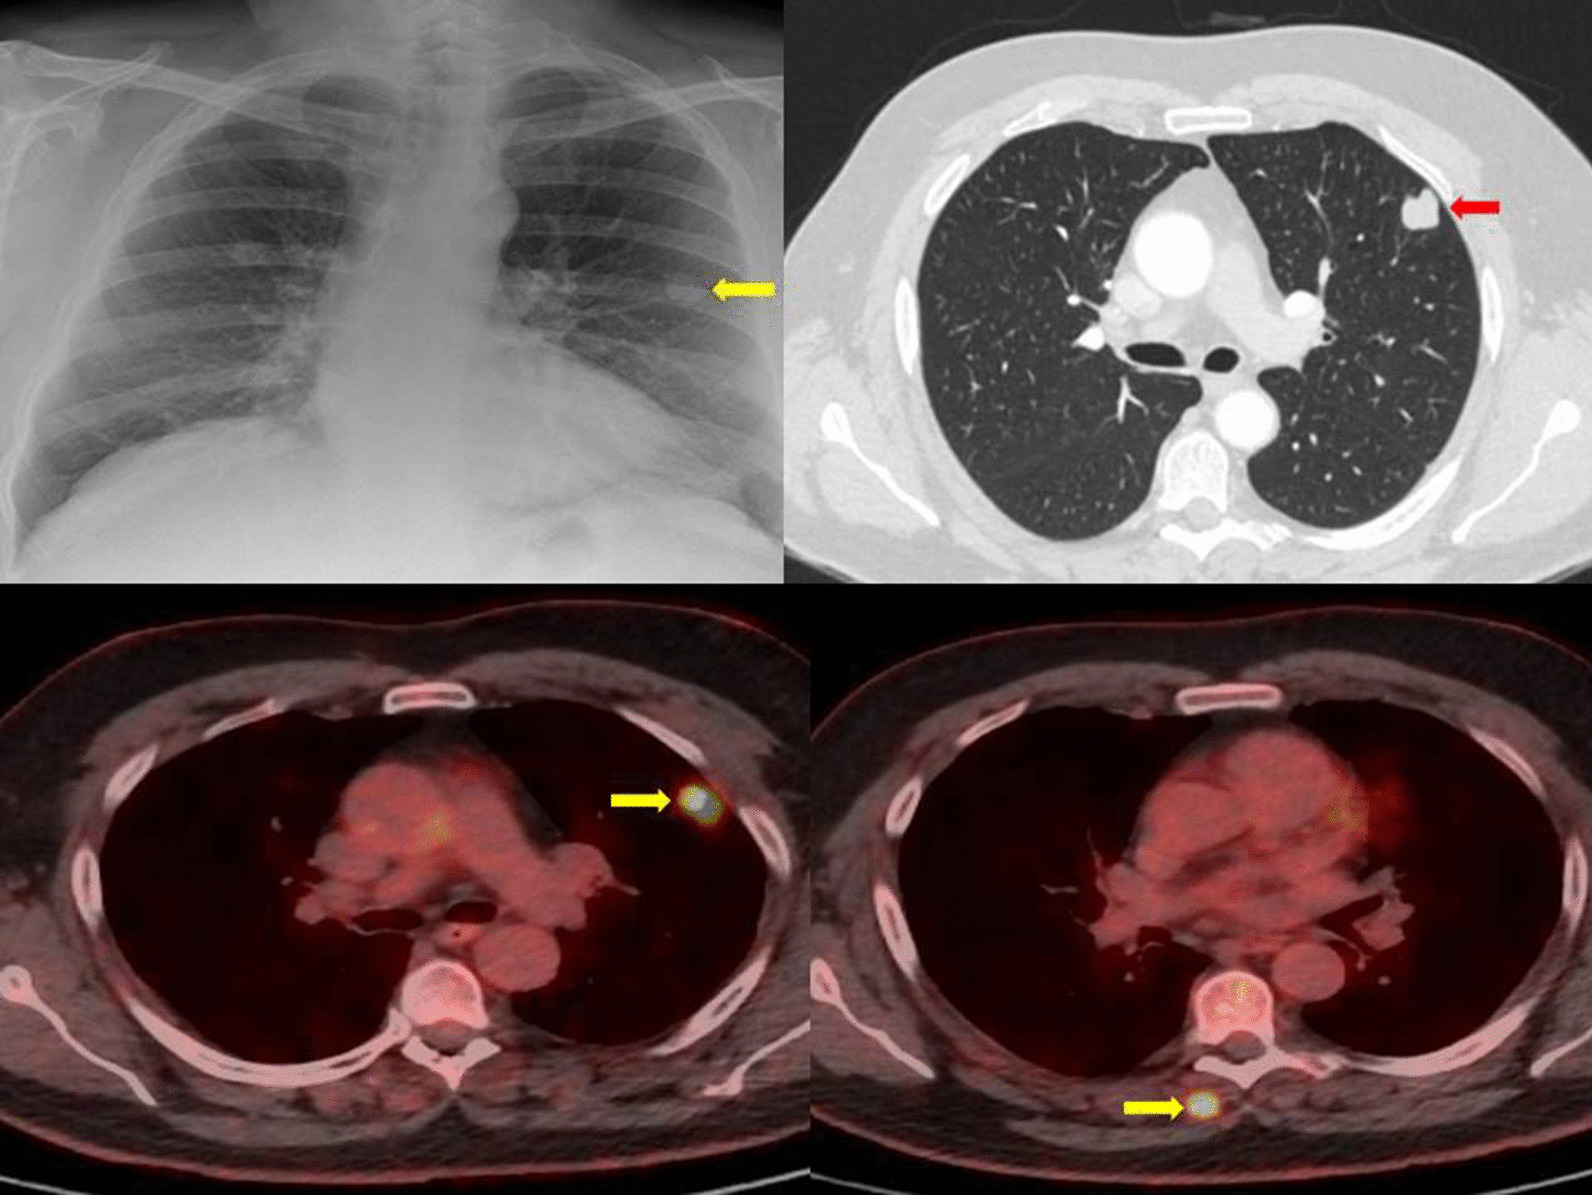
Chest X-ray showing a “coin lesion” in the left mid-zone (yellow arrow). CT section confirming the left-upper-lobe 20 mm solid (red arrow), non-spiculated, solitary nodule. Sections of PET showing high SUV activity of the lung lesion and a separate solitary posterior right paravertebral intramuscular lesion (erector spinae) at the level of T6 vertebral body, not visible on CT (yellow arrows)
